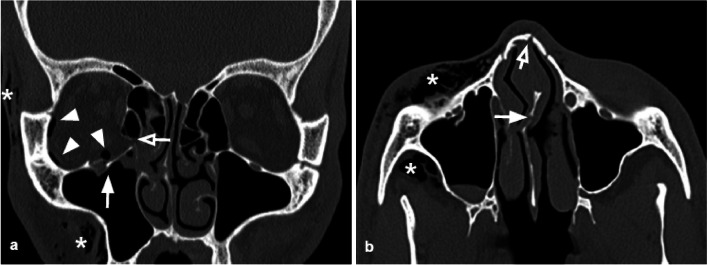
Coronal reconstruction (a) and axial CT acquisition (b) of a Naso-orbitoethmoidal Complex Fracture. Coexistence of a fracture of the right orbital floor with the involvement of the infraorbital groove (white arrow, figure a) and of a fracture of the lower third of the right lamina papyracea (empty arrow, figure a), and of a fracture of the nasal septum (arrow) and of the left nasal bone (empty arrow) in figure (b). Evidence of intraorbital (white arrowheads, figure a) and soft tissue (white asterisks, figure a, b) emphysema
